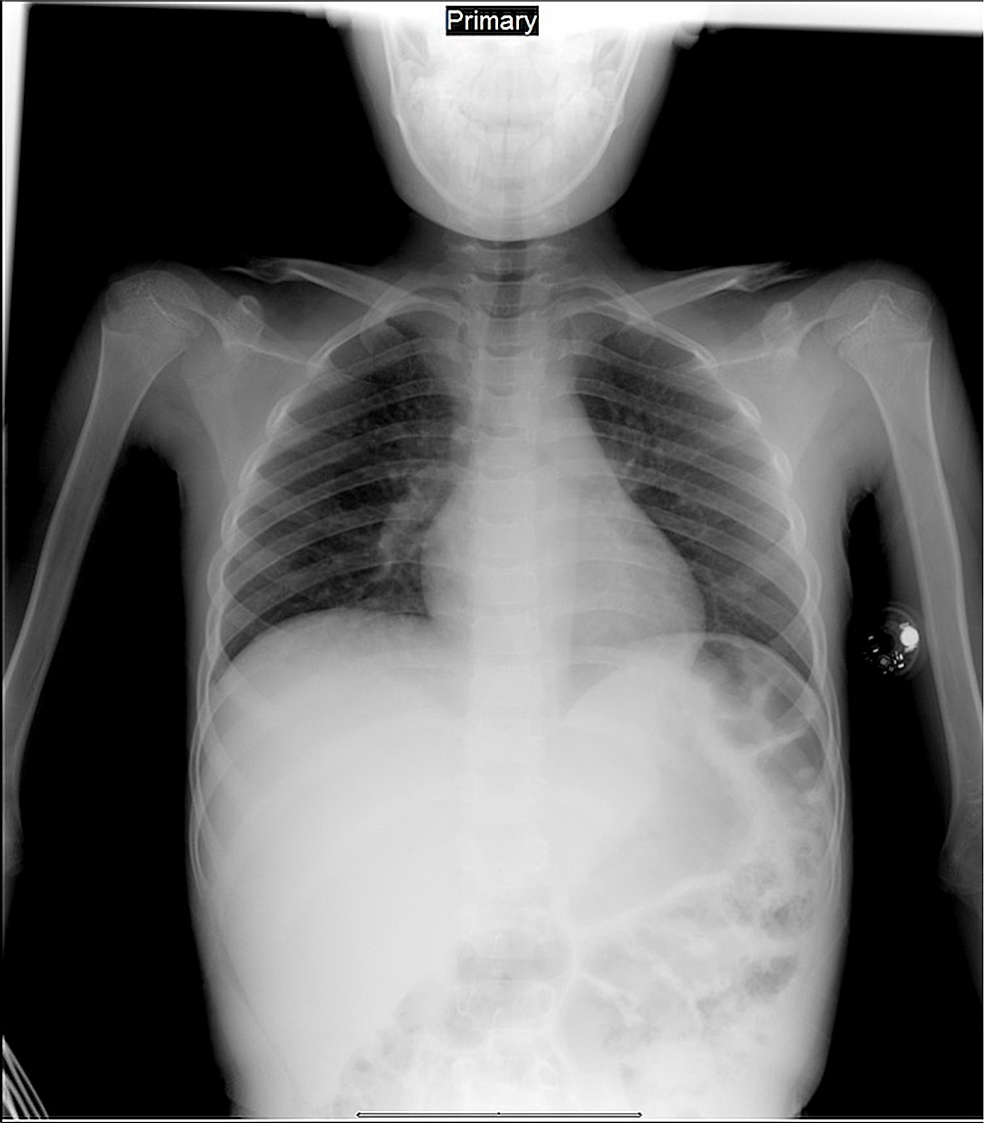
Normal chest X-ray of the patient
